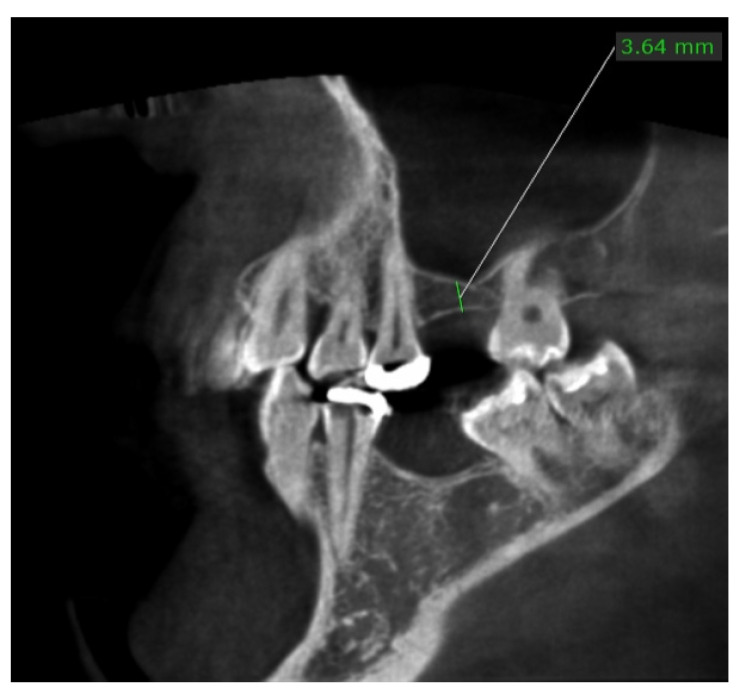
The section of the CBCT showing a residual height of 3.6 mm before the sinus lift at baseline.
